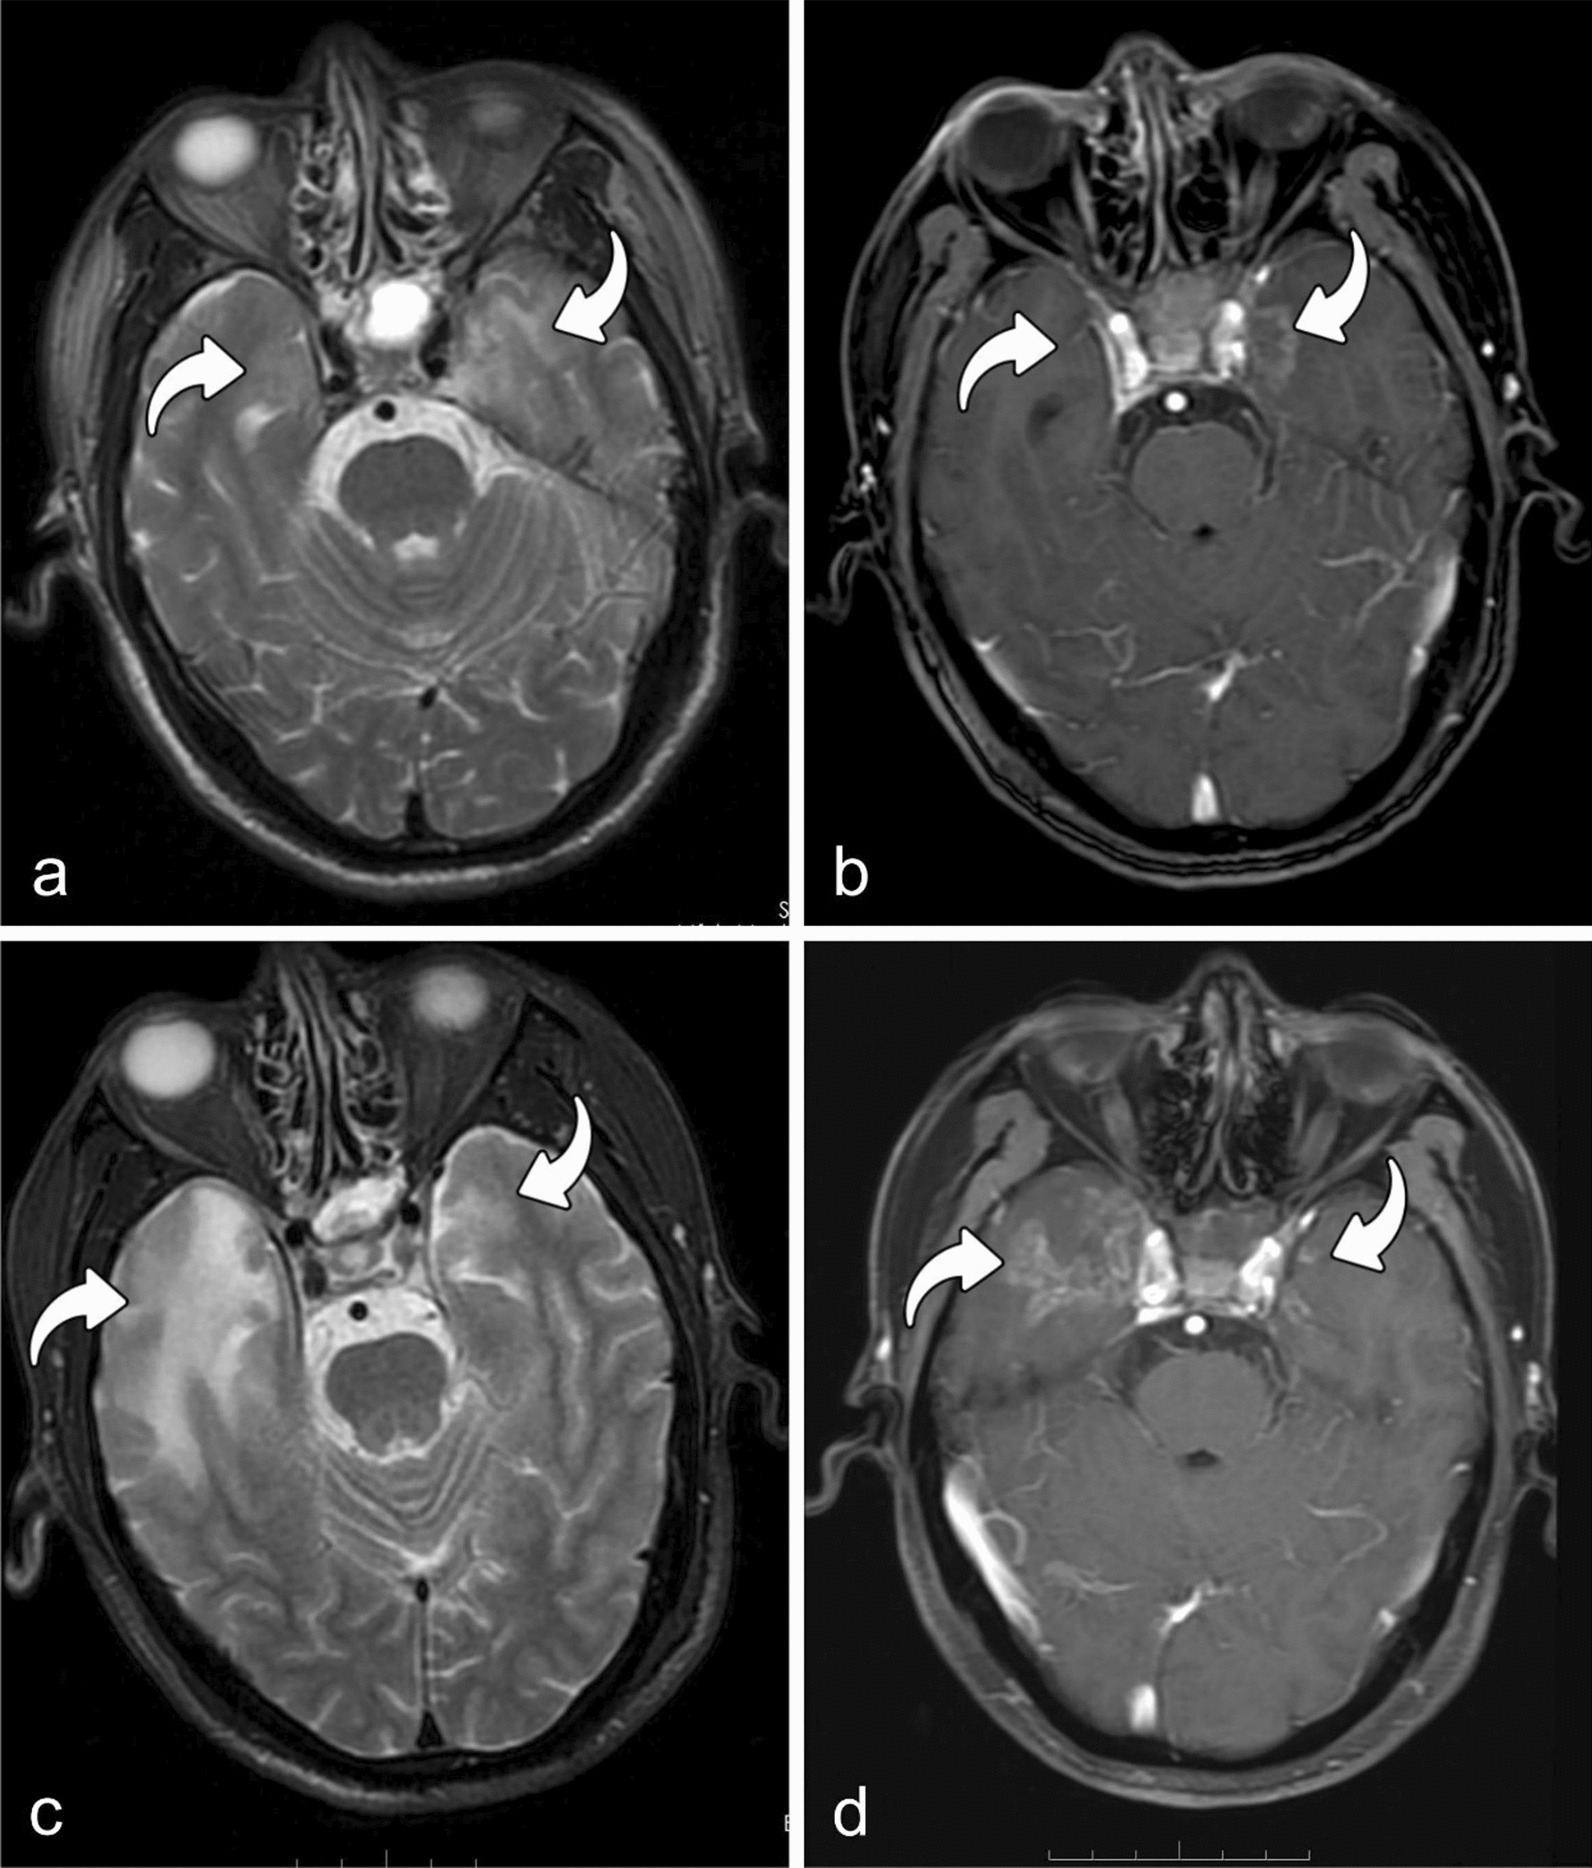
Evolution of bilateral temporal lobe brain injury in one patient (55y, M). Review was conducted at 32.50 months after IMRT. Axial T2-weighted image and post-contrast T1-weighted image show high intensity (a) and significant enhancement (b) in white matter of the bilateral temporal lobes (curved arrow). After 10.03 months, the white matter lesion and contrast-enhanced lesion increased in the right temporal lobe but regressed in size in left temporal lobe (c, d) (curved arrow). *Note*: *IMRT* intensity-modulated radiotherapy
